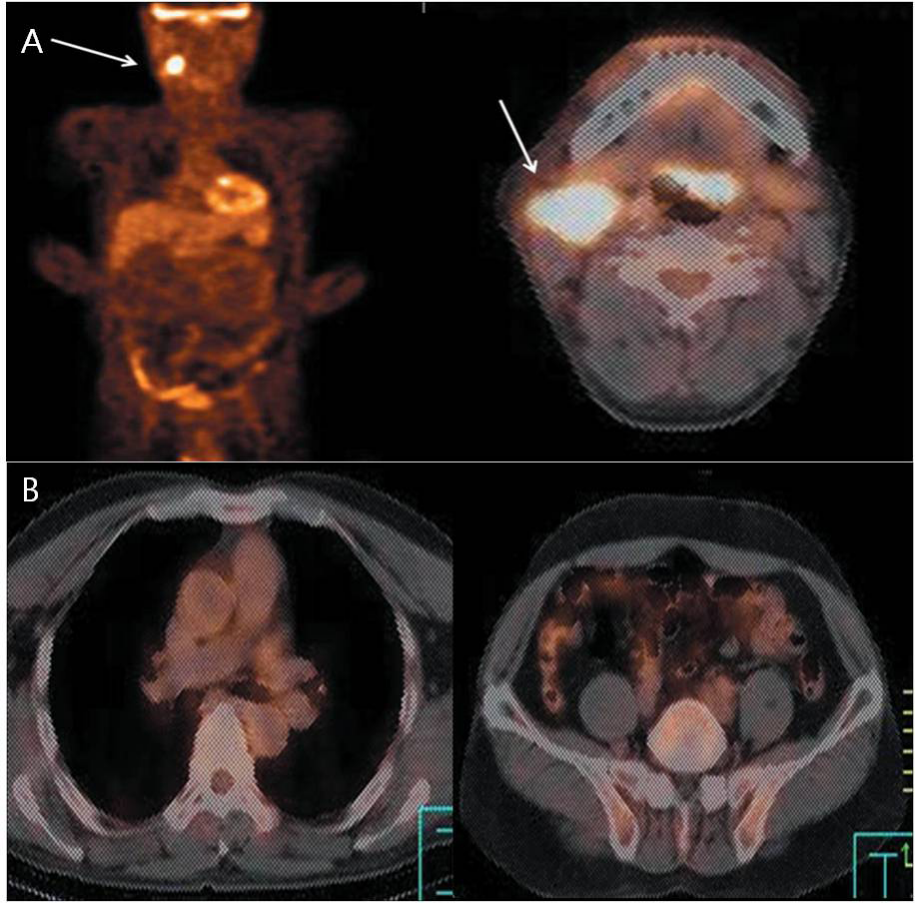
A 54-year-old male with right cervical lymph node metastasis from an unknown primary tumor (patient 9): The patient had radical neck dissection, tonsillectomy, blind biopsy of the nasopharynx and tongue base. The pathology revealed metastatic squamous cell carcinoma in one out of 20 lymph nodes. However, there was no evidence of malignancy in other tissues including tonsiles, tongue base, parotid gland, salivary gland, or nasopharynx; (A) PET/CT showed a hypermetabolic lesion (SUV 13.0) in right cervical lymph node(arrow) at level II; (B) There was no additional FDG uptake suggesting a primary site in the transaxial PET/CT scans of the chest and pelvis.
